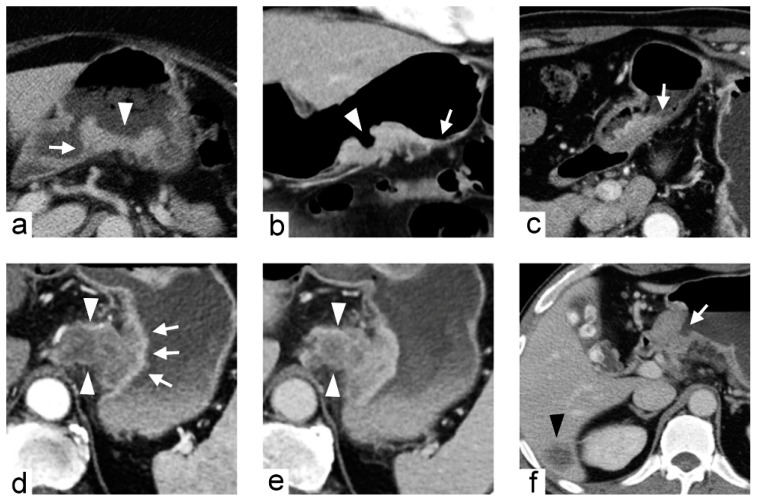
Imaging traits of gastric cancers demonstrated in different patients. (a) 71-year-old female with gastric cancer at posterior wall of gastric body. Contrast-enhanced computed tomography (CT) during portal venous phase axial image demonstrates the tumor with fungating shape (arrowhead), well-defined margin and acute tumor transition angle (arrow). (b) 61-year-old female with gastric cancer at greater curvature of gastric body. Contrast-enhanced CT during arterial phase coronal image demonstrates the tumor with ulcerative shape (arrowhead), ill-defined margin and obtuse tumor transition angle (arrow). (c) 66-year-old male with gastric cancer at antrum. Contrast-enhanced CT during arterial phase axial image demonstrates the tumor with polypoid shape, ill-defined margin and obtuse tumor transition angle (arrow). (d,e) 73-year-old male with gastric cancer at cardia. Contrast-enhanced CT during arterial phase (d) and portal venous phase (e) axial images demonstrate the tumor with an inner layer of higher contrast enhancement (arrows in (d)) and an outer extra-gastric portion of heterogeneously lower contrast enhancement (arrowheads in (d,e)). (f) 56-year-old male with gastric cancer at antrum. Contrast-enhanced CT during arterial phase axial image demonstrates the tumor with luminal obstruction (arrow) and liver metastasis (arrowhead).
